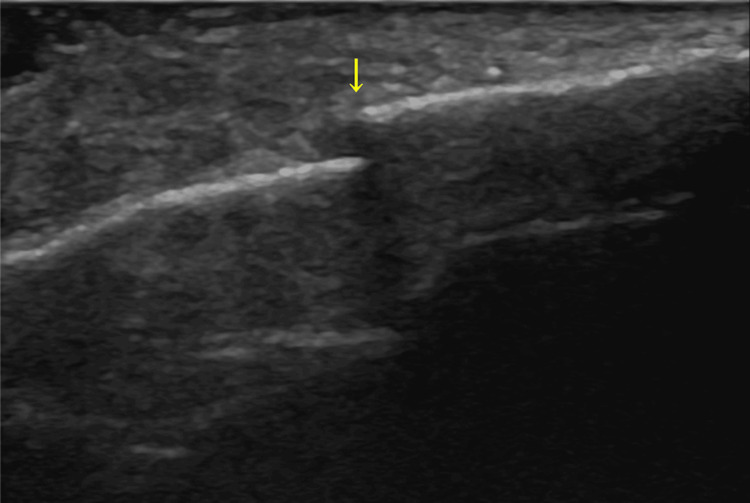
Ultrasound showing a sternal body fracture (yellow arrow).
